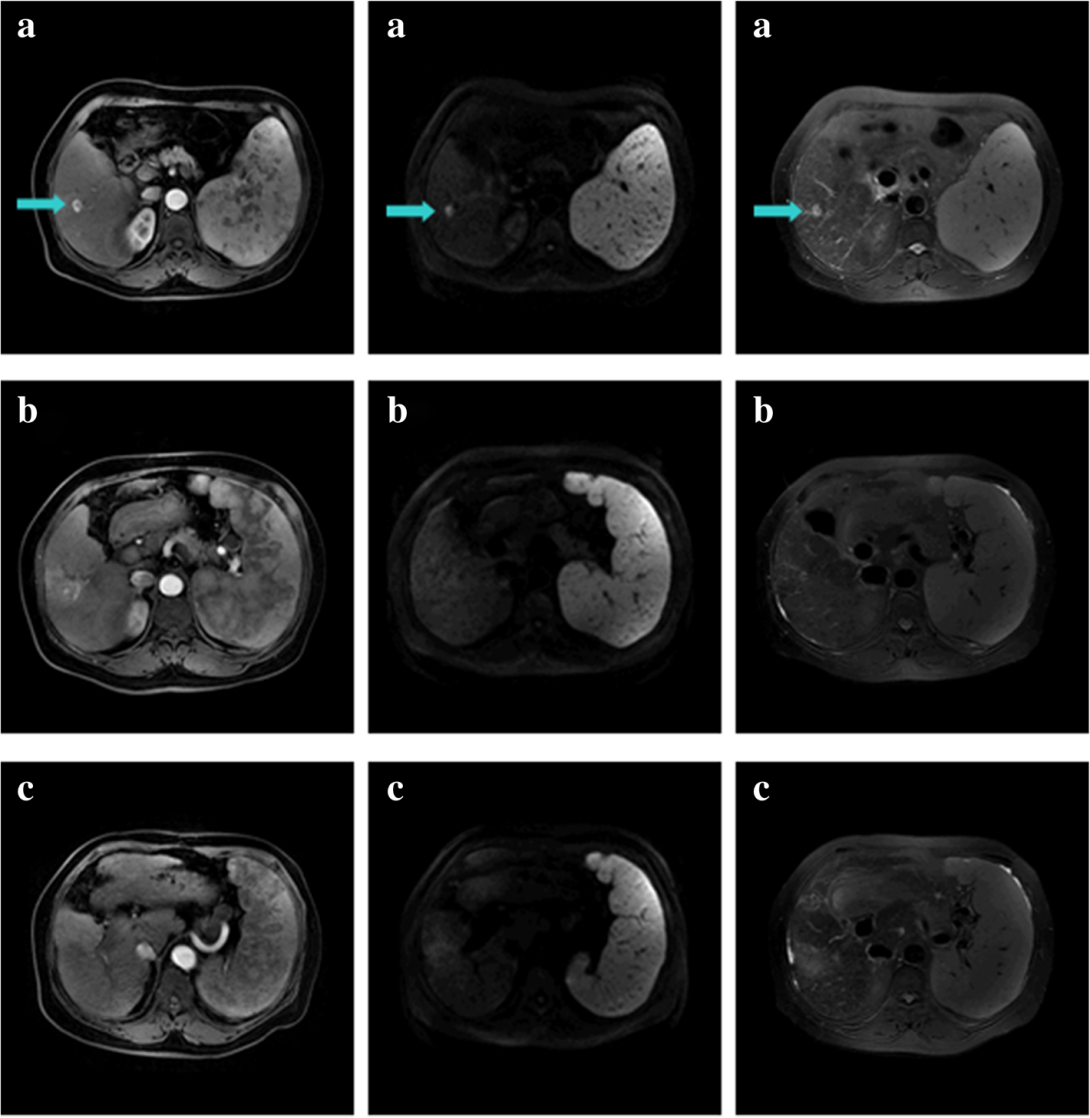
Example of complete response to SBRT assessed by MRI. a The initial abdominal MRI scan with the primary HCC indicated by the arrow. b MRI scan of 6 months after SBRT. This patient was classified as CR at 6 months after SBRT. c MRI scan of 3 years after SBRT. The lesion in liver reveals changes after treatment and no activity
